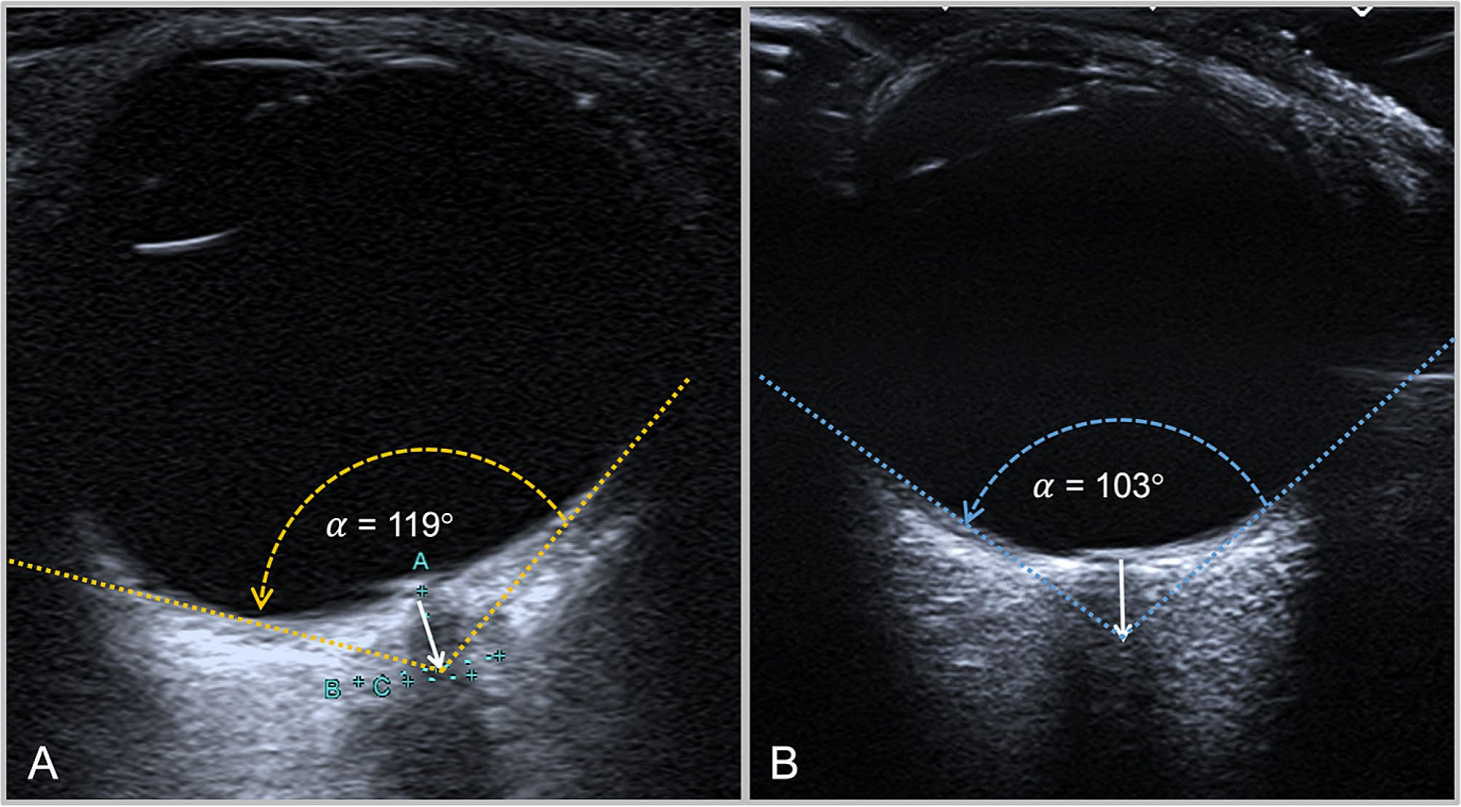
Transorbital ultrasound images of one globe in the axial plane depicting measurements of the PGA at 3.0 mm distance from the ophthalmic lamina cribrosa in a globe with PGF (A) and a normal globe (B)
